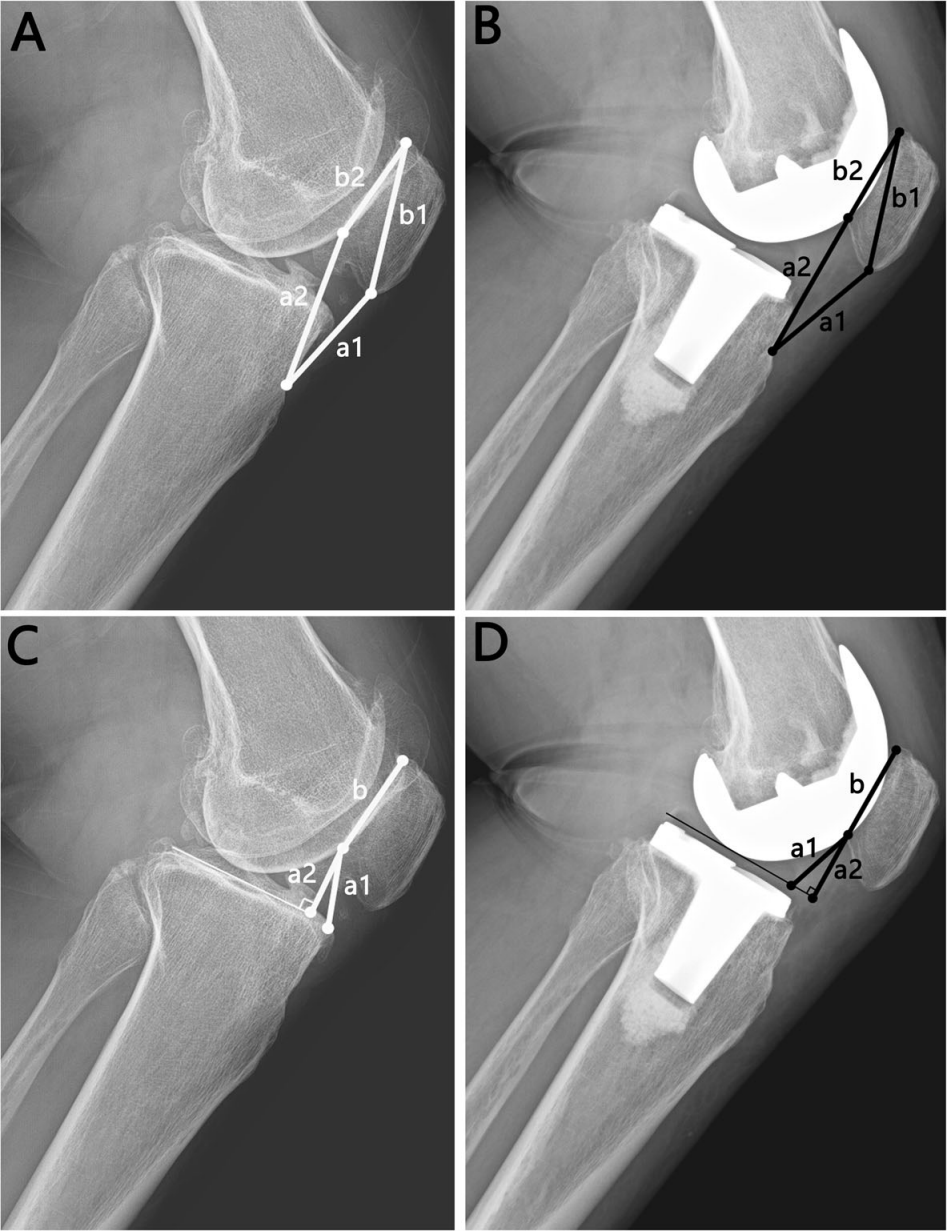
Representation of the four classical methods used for measuring patellar height. As represented in A and B (preoperative and postoperative radiographs, respectively), the Insall-Salvati index is calculated by “a1/b1”, and the modified Insall-Salvati index is calculated by “a2/b2”. As represented in C and D (preoperative and postoperative radiographs, respectively), the Caton-Deschamps index is defined as “a1/b”, and the Blackburne-Peel index is defined as “a2/b”
